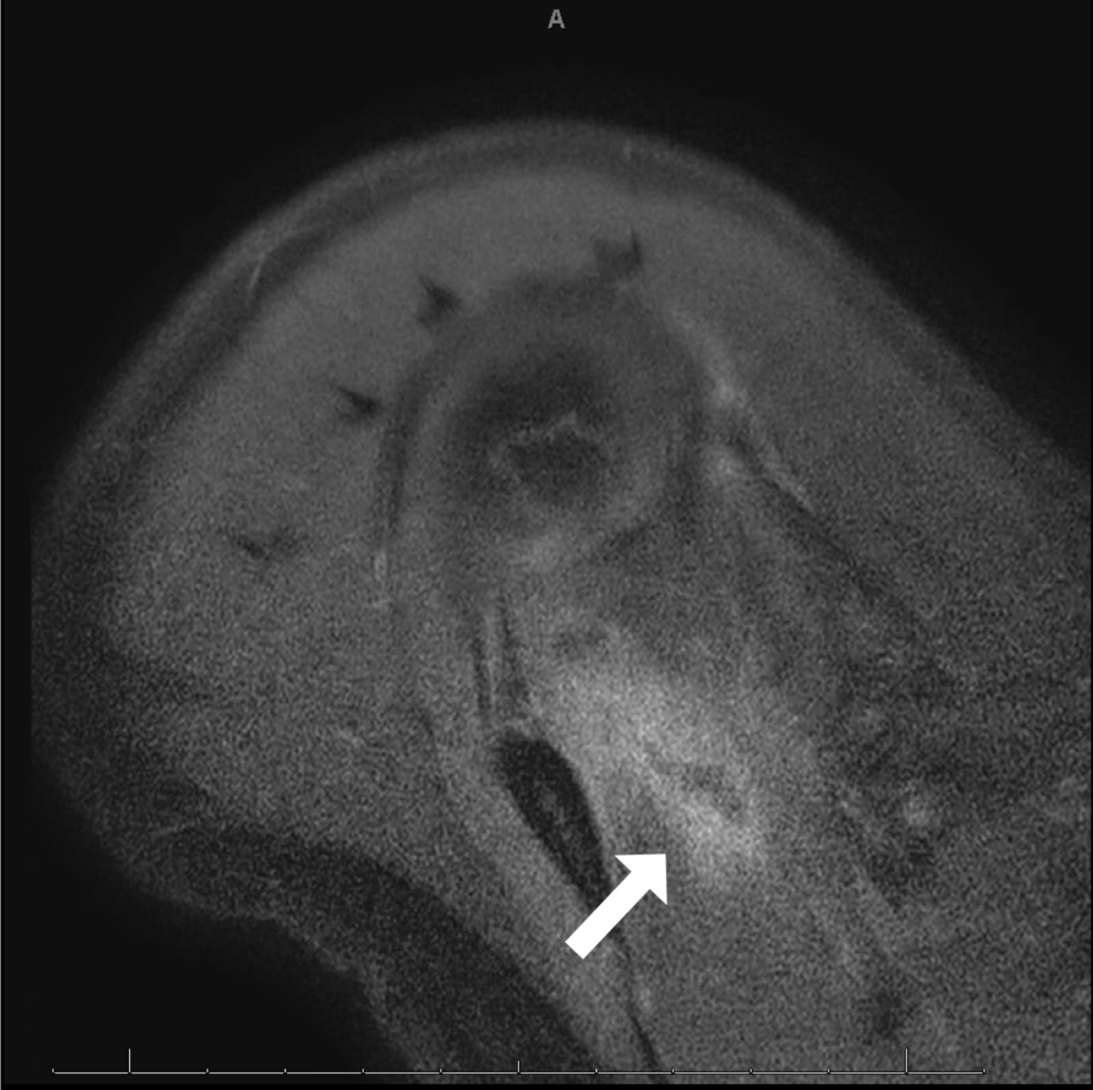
Right axial T1-weighted MRI showing supraspinatus lesion with irregular peripheral edema and non-enhancing central core suggestive of myonecrosis. MRI, magnetic resonance imaging
